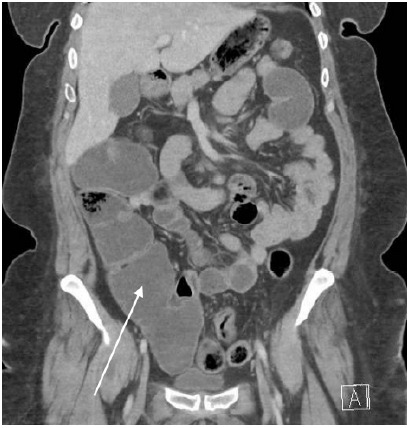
Computed tomography of the abdomen and pelvis (coronal) demonstrating dilated, fluid-filled large bowel/ascending colon (arrow) without obvious obstruction or transition point.
